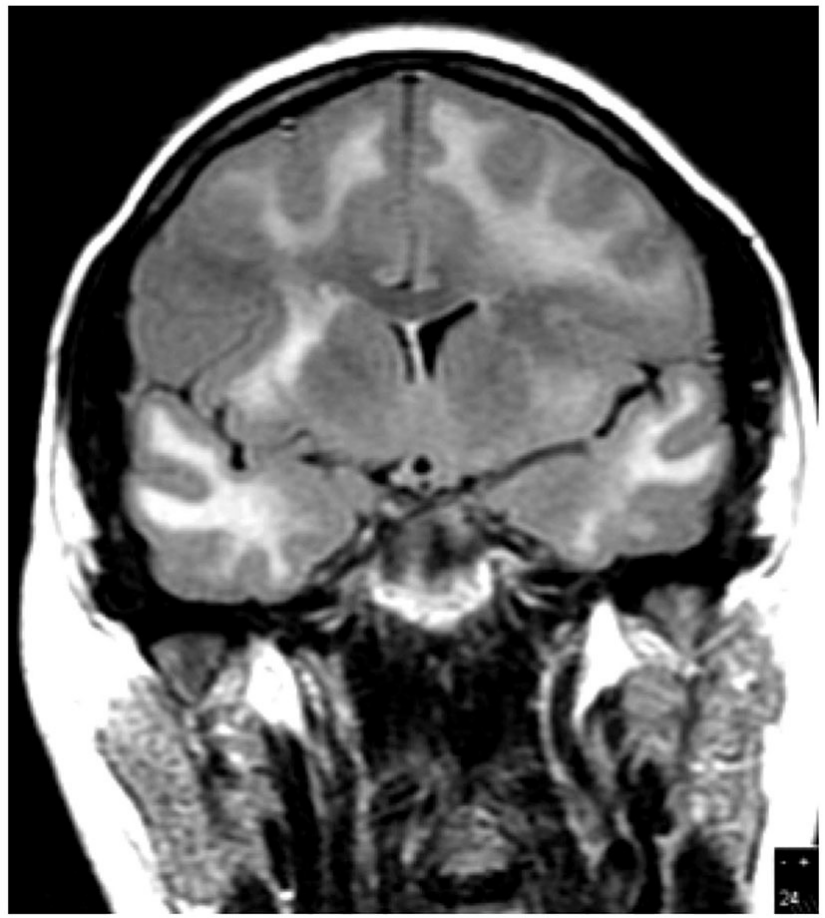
Case 7: Brain MR imaging (coronal T2-weighted image) demonstrating extensive white matter hyperintensity.
